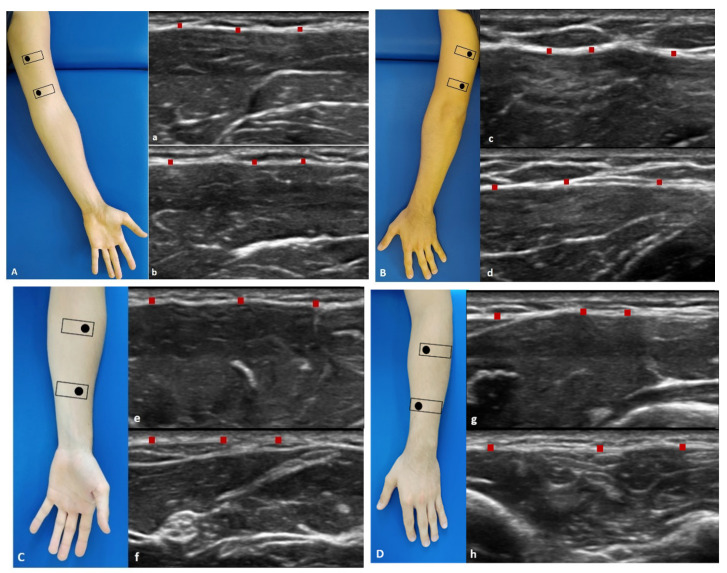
Ultrasound (US) images of: the anterior region of the arm (A) and of the forearm (C); the posterior region of the arm (B) and of the forearm (D). Anterior region (A,C) at the levels Ant 1 (a,e) and Ant 2 (b,f). Posterior region (B,D) at levels Post 1 (c,g) and Post 2 (d,h). Probe: black rectangle. Red dashes: deep fascia.
